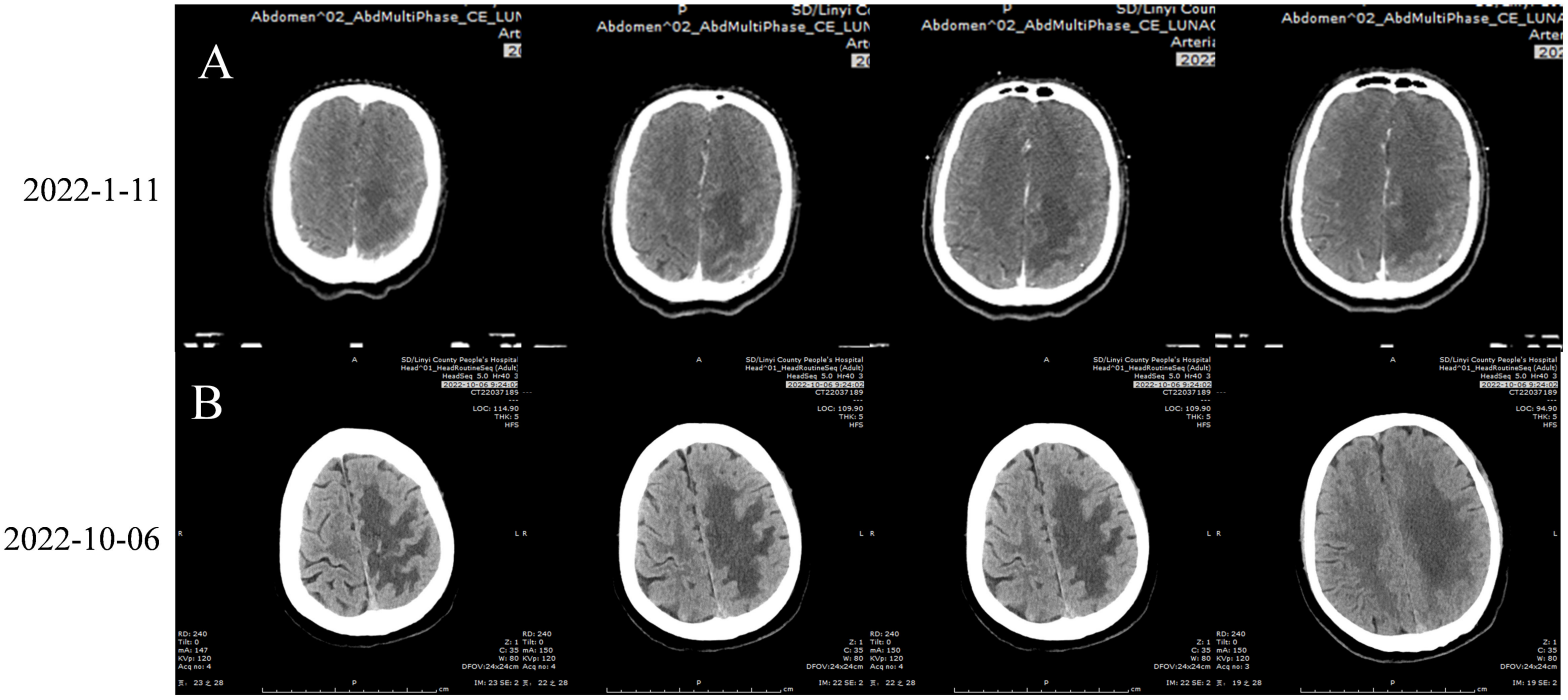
(A) 2022-1-11 Brain Enhanced CT: A circular lesion with a size of about 1.6×1.8cm was observed at the junction of gray matter in the left parietal lobe, with large sheet edema surrounding the lesion and a centered midline structure. Irregular bone destruction on the left parietal bone. (B) 2022-10-6 Brain Enhanced CT:A circular lesion with a size of approximately 1.4 × 1.6cm can be seen in the gray white border area of the left parietal lobe. The lesion is surrounded by large areas of edema, and the midline structure is centered. Irregular bone destruction area was observed in the left parietal bone, with no significant changes compared to before (January 11, 2022). Figure of Timeline. Timeline of treatment course of case reports. PR, partial reaction; PD, disease progression.
